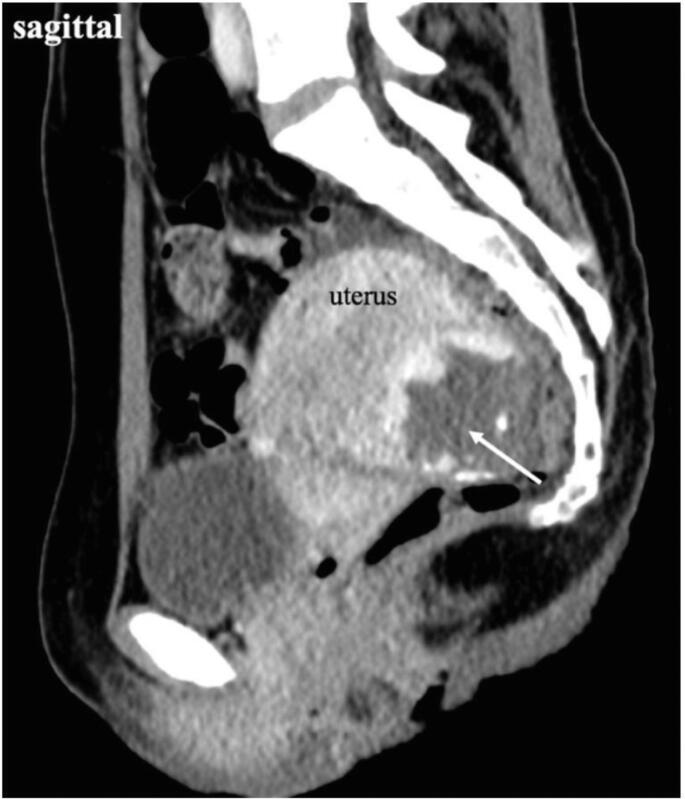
Sagittal contrast-enhanced CT scan of the pelvis demonstrates a mass posterior to the uterus (arrow). The mass shows marked enhancement at the periphery and was suspected to be a gestational sac implanted in the peritoneum.
